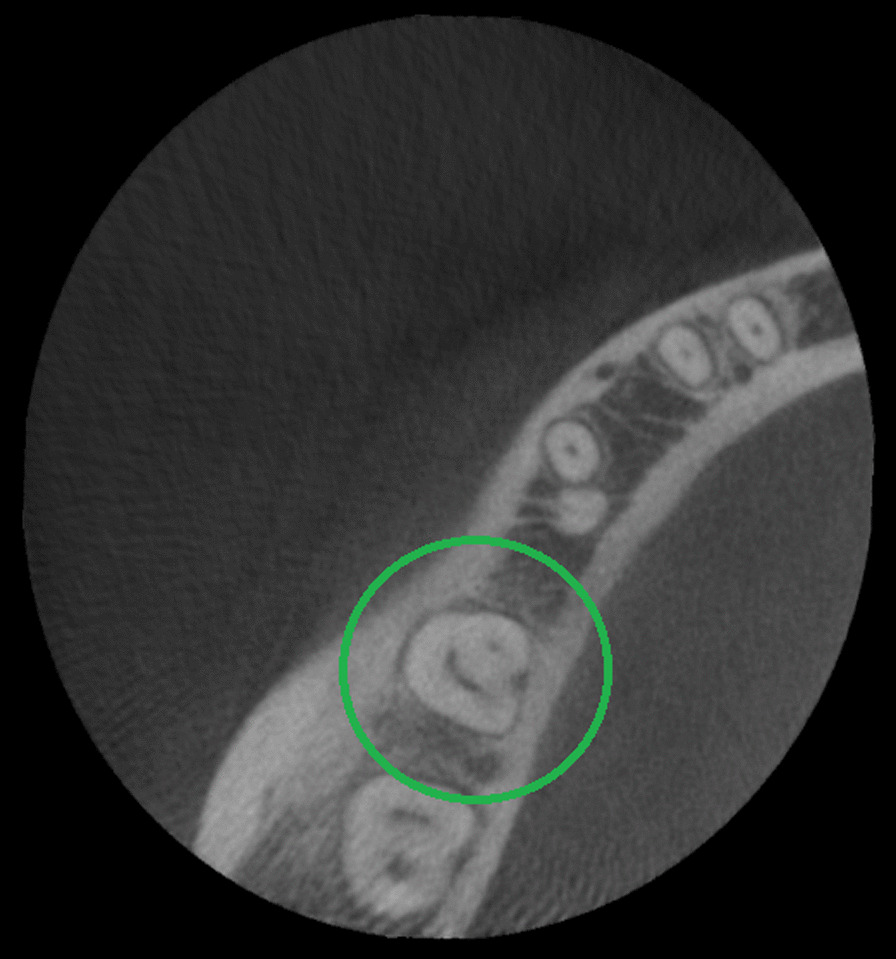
Axial view from CBCT scan showing mandibular second molar with C-shaped root canal system configuration C2 according to Fan et al. [4]
